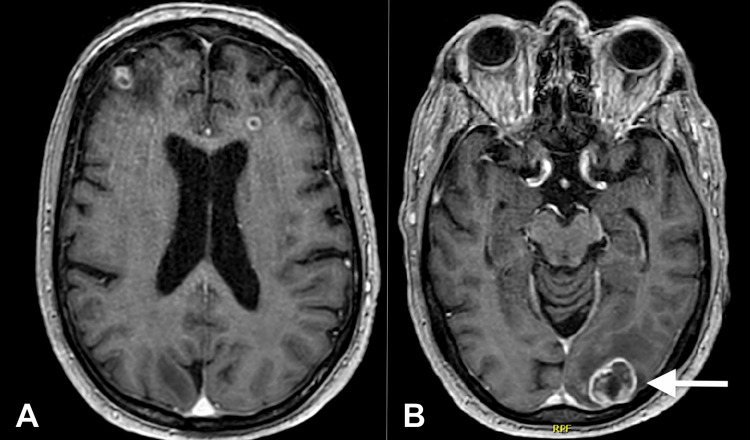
Brain MRI was performed with and without gadolinium contrast. Images displayed (A) and (B) represent post-contrast sequences demonstrating multiple supratentorial heterogeneously enhancing and ring-enhancing cortical-based lesions, some demonstrating internal blood products. Findings are highly concerning for hemorrhagic intracranial metastases. The largest metastasis is within the left occipital lobe, measuring 2.5 × 2.1 × 2.1 cm (B, white arrow). Vasogenic edema is seen surrounding the intracranial metastases and is most pronounced within the left occipital lobe. RPF: Reference position frame
